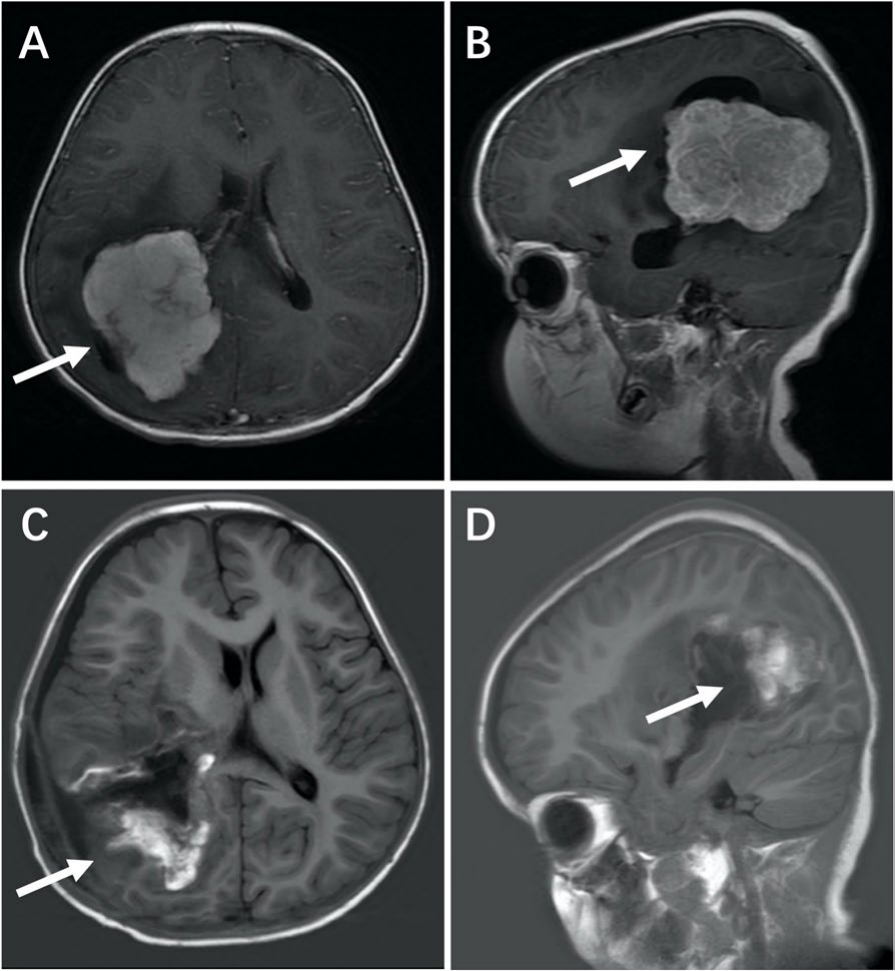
Brain MRI before the operation; (A, B) A giant (4.9cm*7.0cm*5.9cm), irregular, lobulated and space-occupying lesion in right parietooccipital lobe. Brain MRI after the operation; (C, D) No residual tumor in brain
